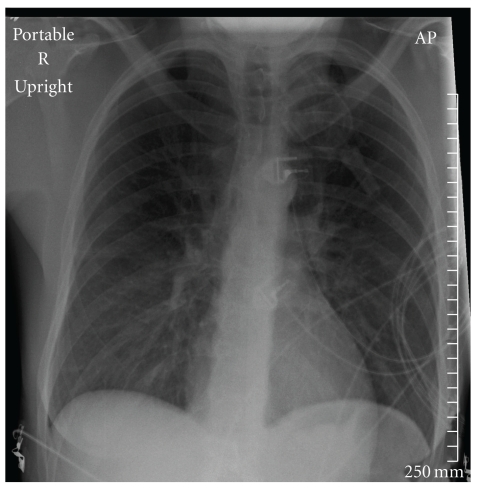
Case 2 chest X-ray at time 1944. Chest X-ray taken two hours after admission shows slight ground glass abnormalities despite significant improvement.
